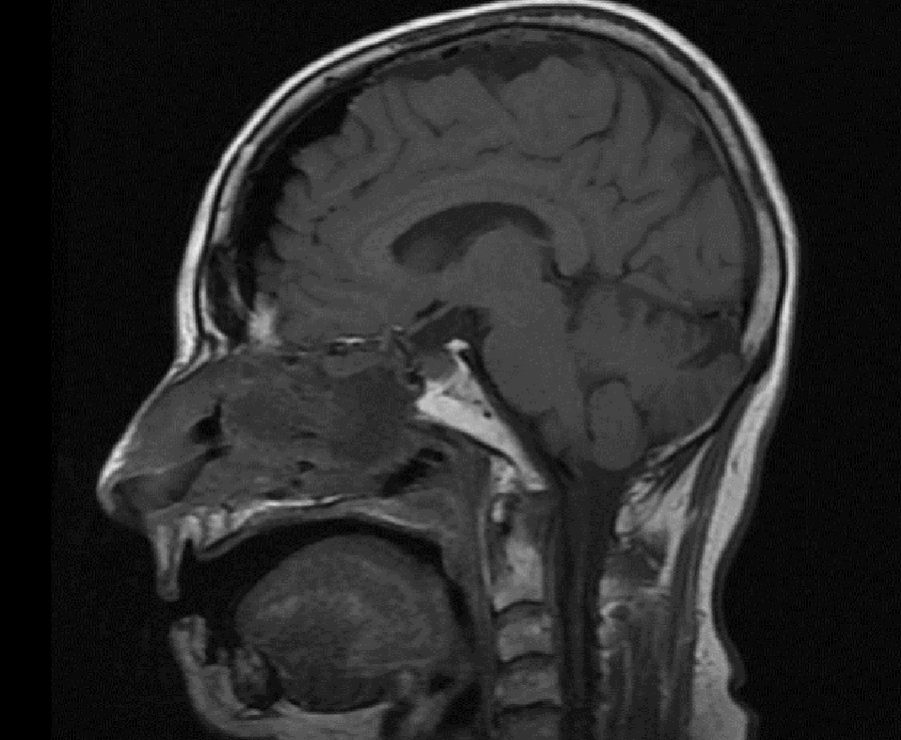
Postoperative sagittal T1 MRI shows gross total resection of the tumor
